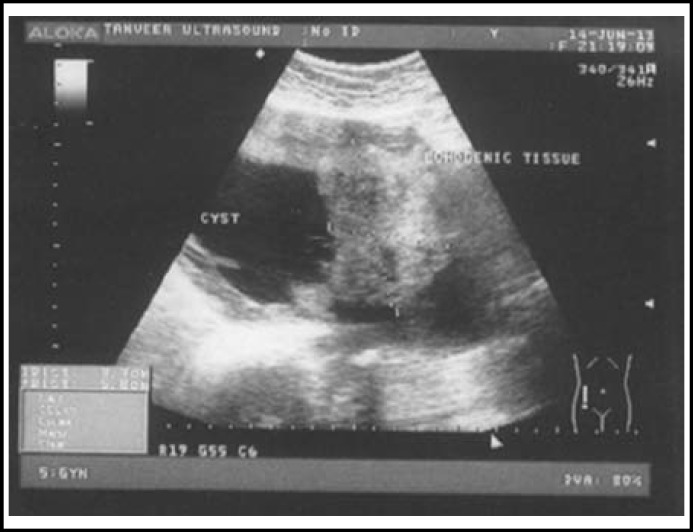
Ultrasound showing a large solid cum cystic mass of 19.7x16.8x13.7 cm with multiple thin septae inside the cyst
